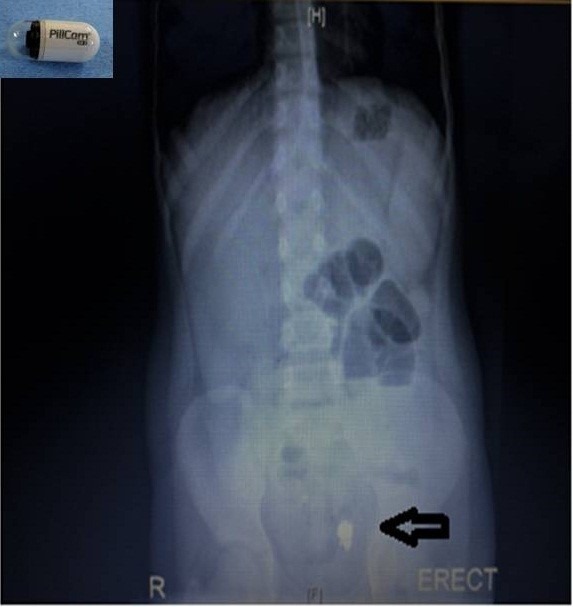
Figure 1:X-ray abdomen showing the retained capsule. Inset—PILLCAM^TM^ SB3 capsule system.
